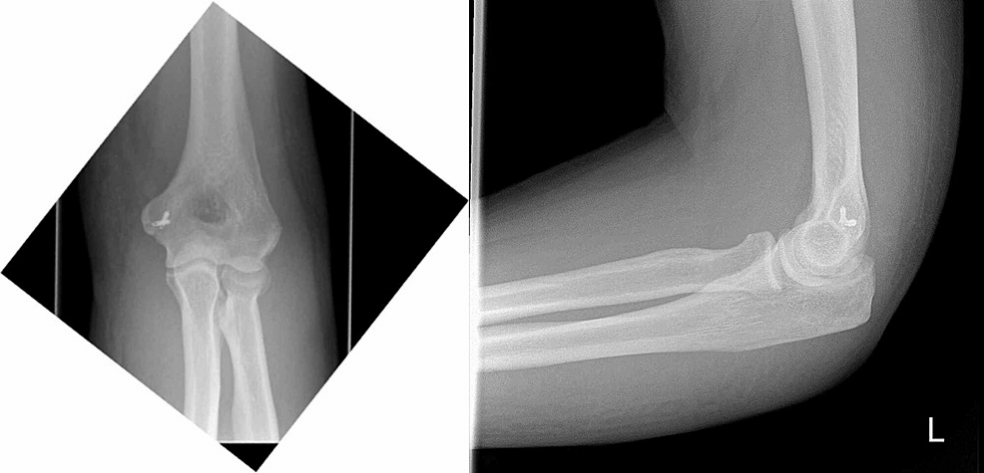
AP and lateral radiographs of elbow postoperatively demonstrating the position of suture anchors. AP, anteroposterior
